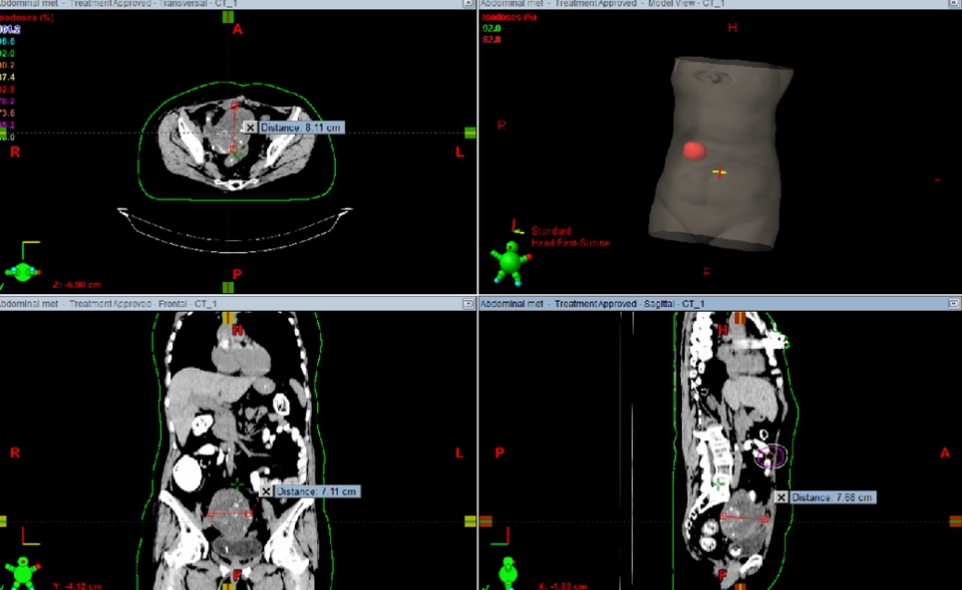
Abdominal CT scan follow-up A decrease in size of the abdominal mass treated previously. The volume of the lesion has decreased from 1495 cc to 442.8 cc (over 70%).
